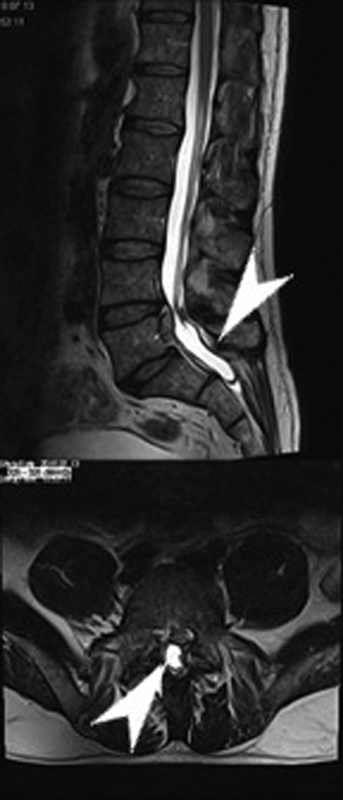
A sagittal and axial T2-weighted magnetic resonance imaging obtained before admission demonstrating a left-sided herniated disc at the L5 to S1 segment (arrowheads).
